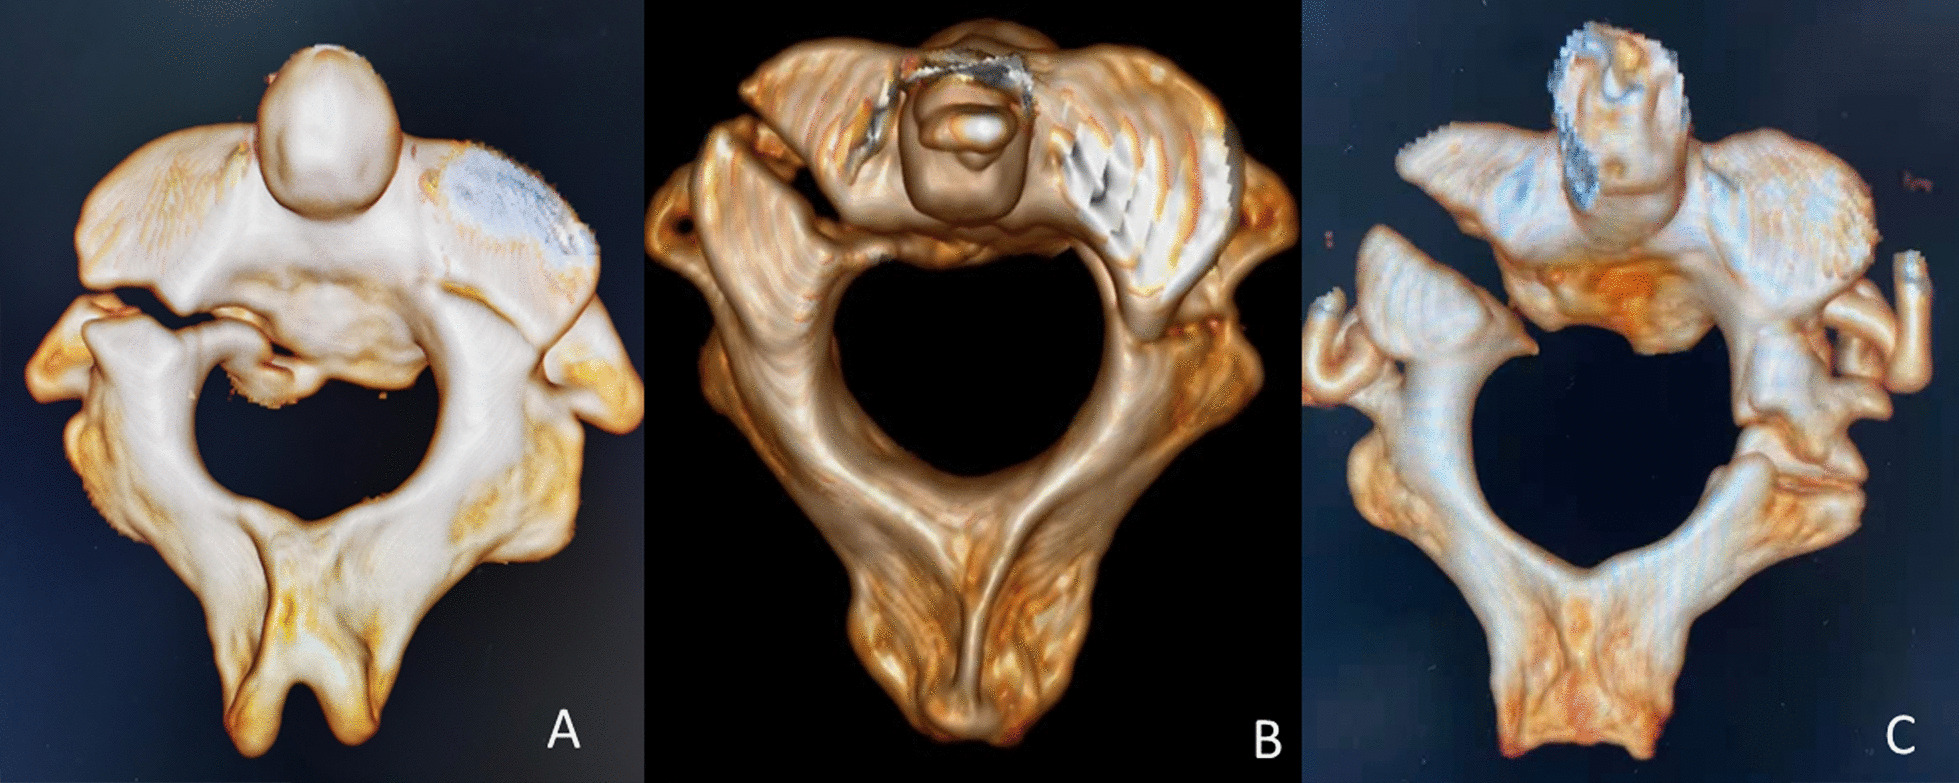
Three-dimensional reconstruction CT image showed injuries of anterior element on one side and the other side injury located in the anterior, middle or posterior element: A two anterior elements of C2 ring, with left fracture line extending from superior articular process to posterior vertebral wall and right fracture line through superior articular process; B anterior and middle elements injuries, with left fracture line through posterior wall and upper articular surface of C2 and right PIC fracture; C anterior and posterior elements injuries with left fracture line through posterior wall and upper articular surface of C2 and right inferior articular process fracture
